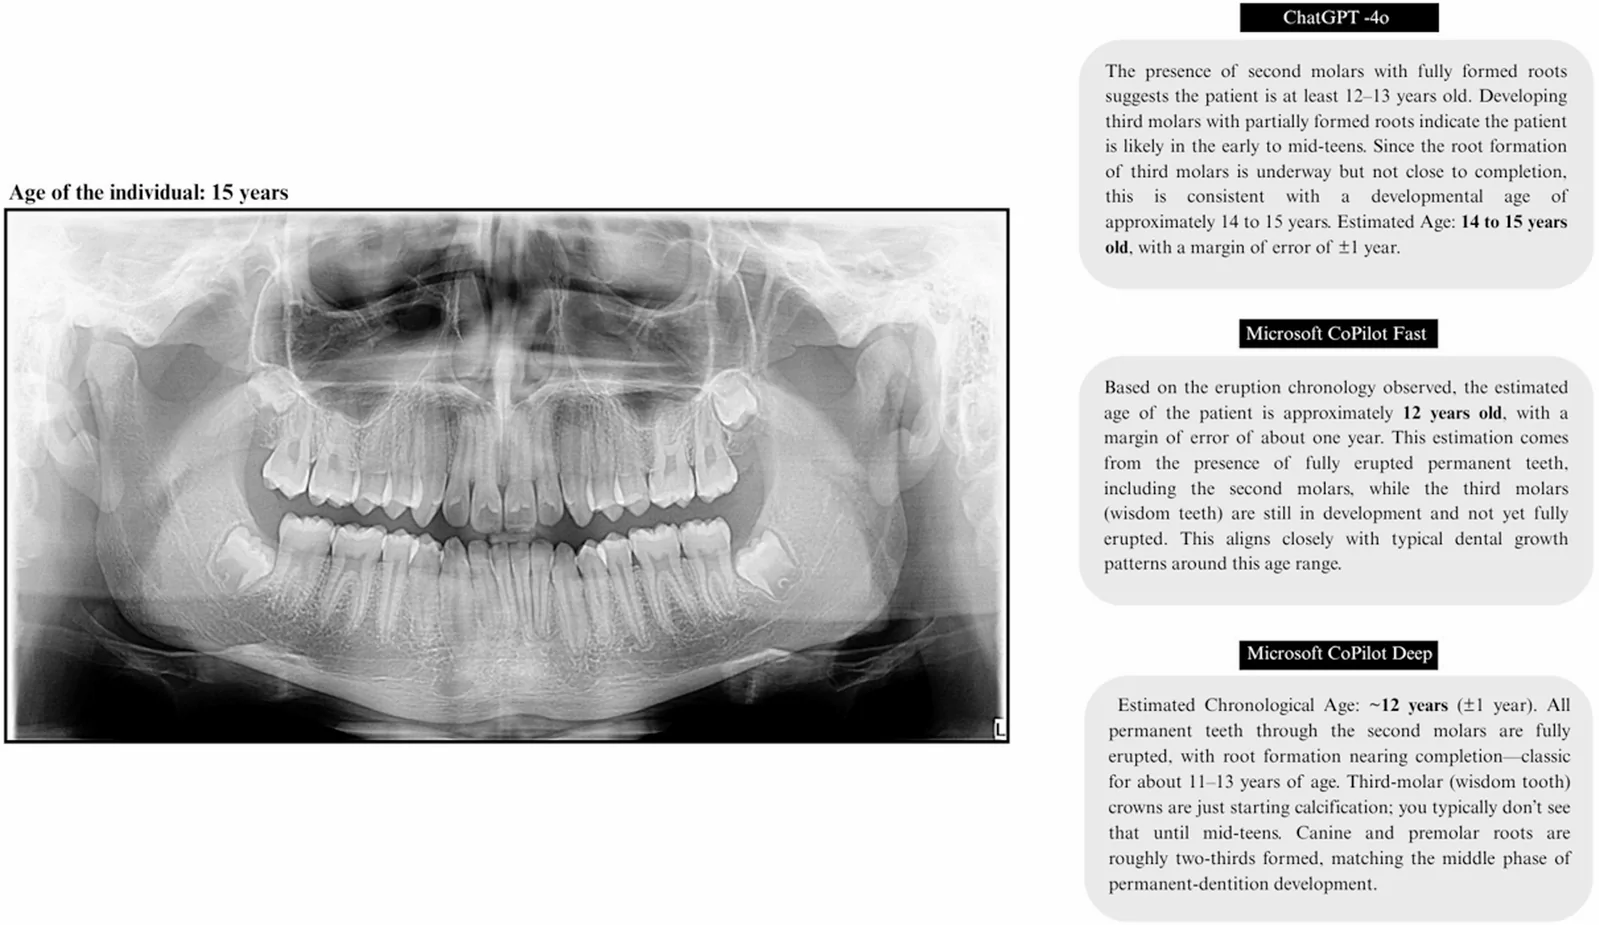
Example of full responses generated by the VLMs (ChatGPT-4, Microsoft Copilot Fast and Microsoft CoPilot Deep), based on panoramic radiography from a 15-year-old individual. The estimated age provided by each model is highlighted in bold within the output
